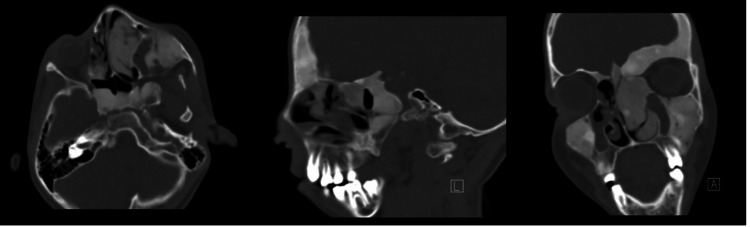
Fibrous dysplasia Plain CT scan of the paranasal sinus showing hyperostosis of the skull and facial bone with areas of sclerosis and ground-glass appearance. Biopsy was taken only from the inferior turbinate, confirming the diagnosis.
